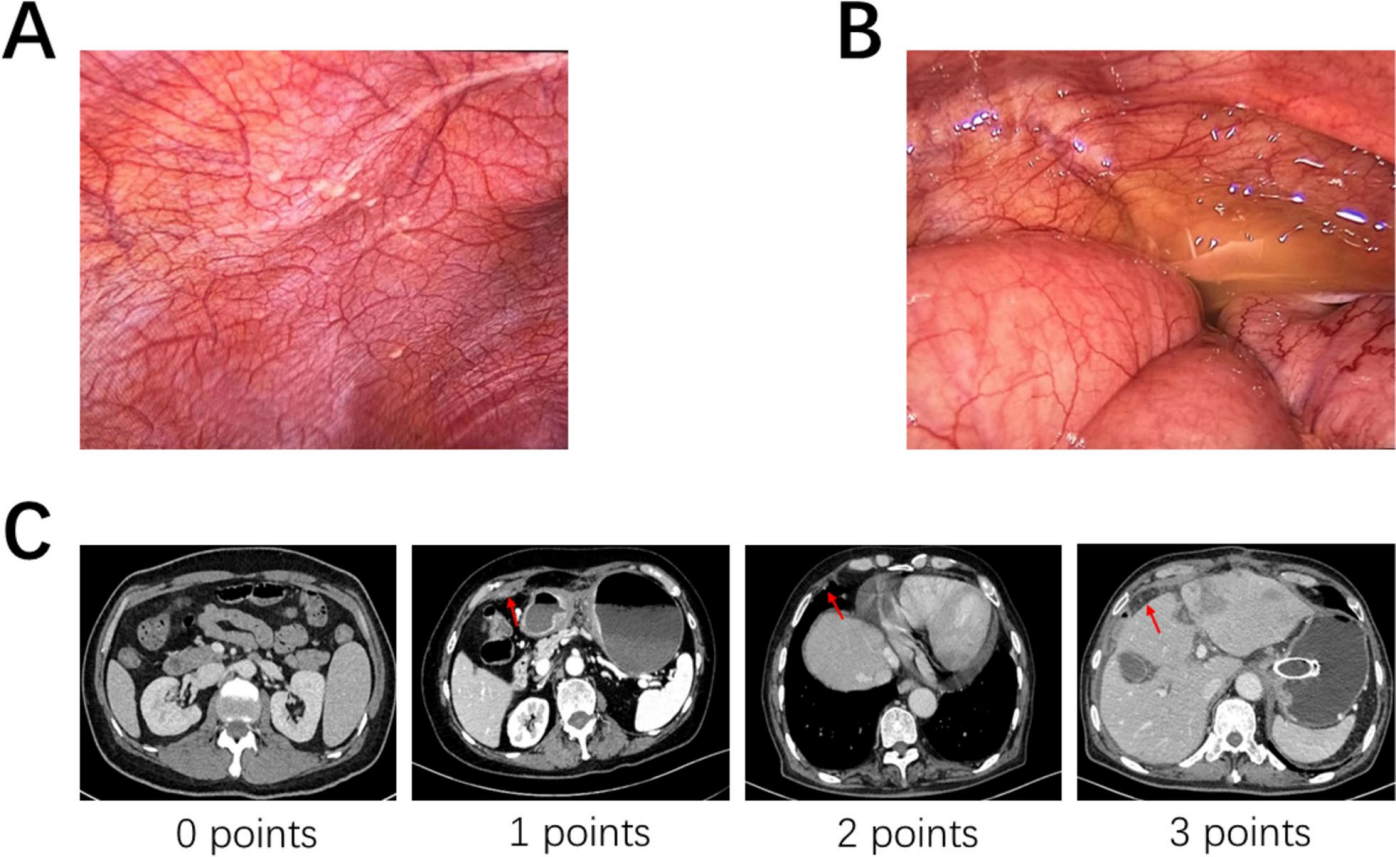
Endoscopic exploration and occult peritoneal metastasis (OPM) score of the peritoneum attached to the organs or tissues. A Peritoneal malignant nodules. B Malignant ascites in the abdominal cavity. C Computed tomography (CT) findings that correspond to the aforementioned criteria. The degree of metastasis is displayed from light to severe
